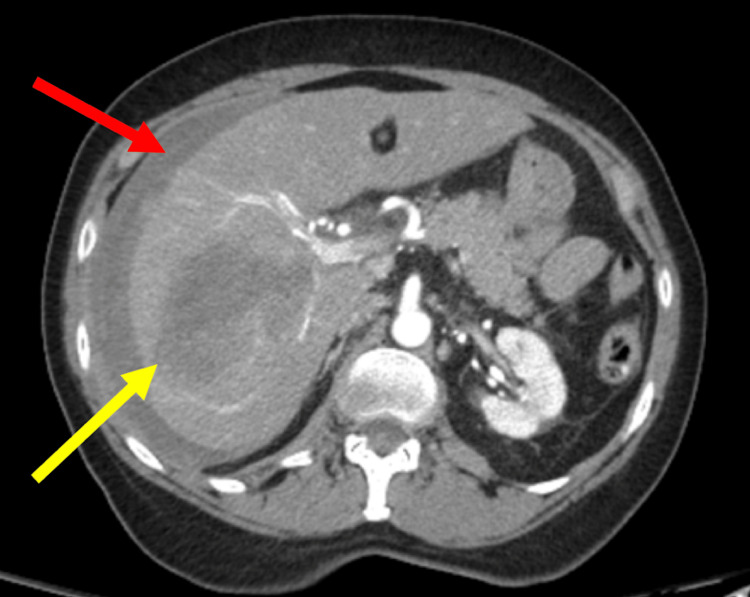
CT scan of the abdomen and pelvis with angiography with a transverse view showing a large hypodense area in the liver (yellow arrow) with disruption of the liver capsule and acute hemoperitoneum (red arrow) without active arterial contrast extravasation. CT: computed tomography.
